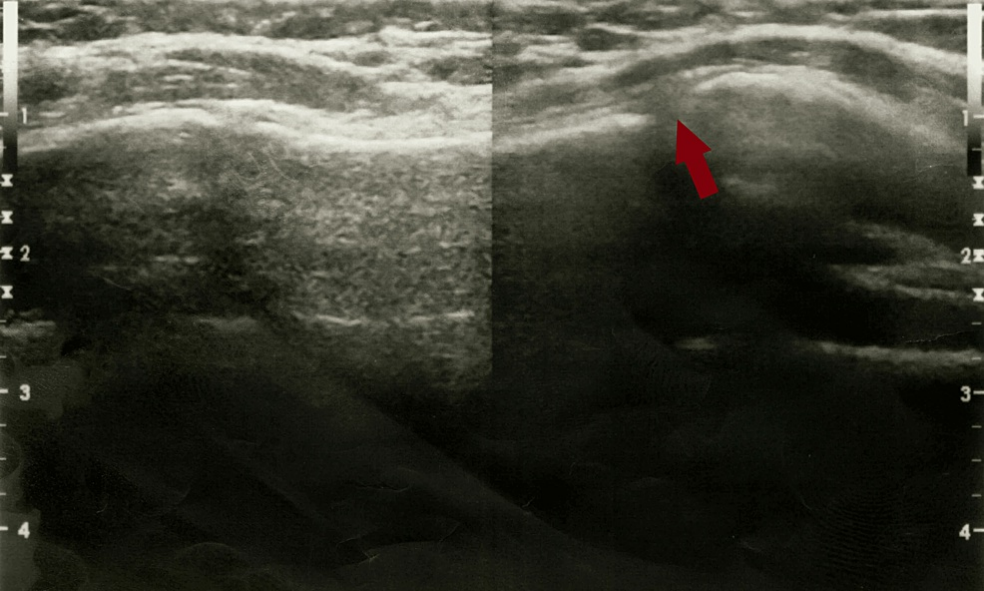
Ultrasound scan image of the sternocostal joints done during the first visit Ultrasound scan image of the sternocostal joints at the first visit shows an oval-shaped hypoechoic focus which is typical for Tietze syndrome.
